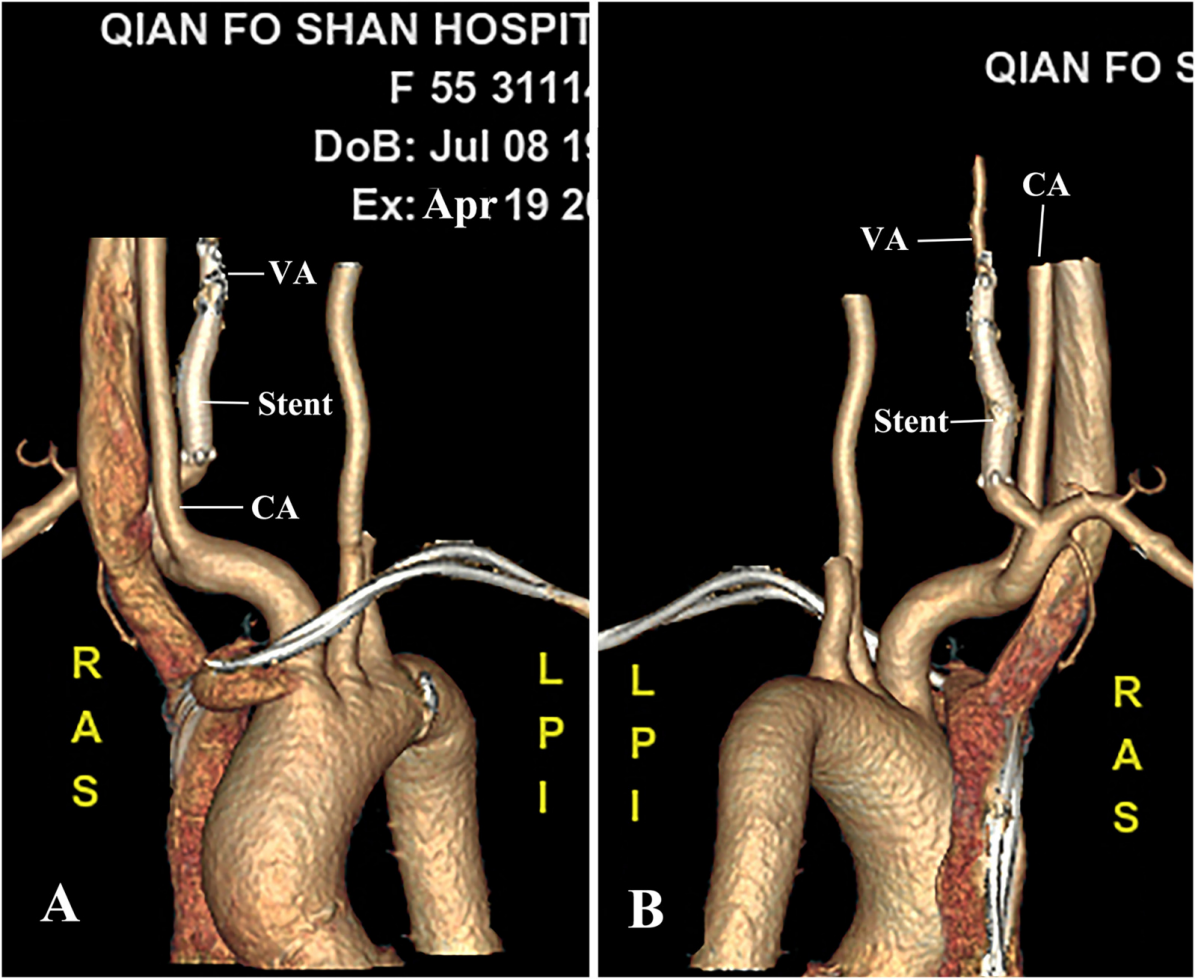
CTA at 6-month follow-up. (A,B) Better luminal filling of the VA was observed on CTA without intra-stent stenosis and recurrence of the fistula or pseudoaneurysm. CA, carotid artery; VA, vertebral artery.
